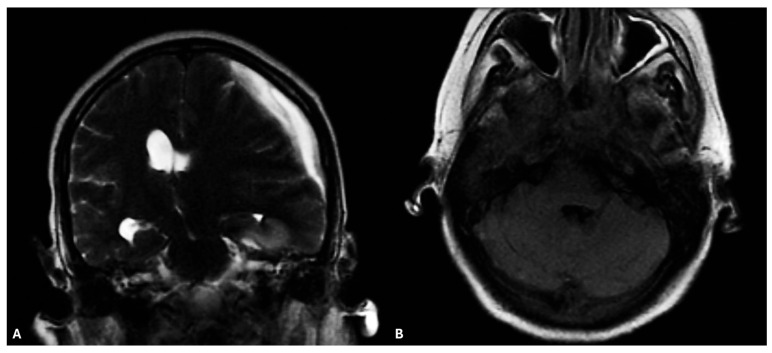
(A) Brain RM revealed Left hemispherical supratentorial subdural fluid layer, without hypointense hemorrhagic components in SWI in context, with a maximum thickness of 17 mm (vs. 14 mm), presenting with peripheral contrast enhancement and diffusion restriction, similar to empyema. Increased mass signs with compression of the left ventricle, and an 11 mm (vs. 9 mm) displacement of the septum pellucidum to the right, with apparent left uncial progression. Right ventricular dilation (due to incarceration), with T2W/FLAIR hyperintensity at the level of the posterior horn, in a context of hydrocephalus due to obliteration of the foramina of Monro. (B) Evaluation of the facial structures demonstrated extensive mucosal edema involving the sphenoidal sinuses and ethmoidal labyrinths, suggestive of an underlying sinonasal infection with possible contiguous spread to the intracranial compartment. No signs of acute intracranial hemorrhage or hydrocephalus were observed. These findings raised concern for cerebritis and incipient subdural empyema. Technique: 1.5 T scanner, FSPGR, FSE, SWAN, 3D-FLAIR, and DWI sequences in multiple planes before and after intravenous administration of paramagnetic contrast medium (ProHance 0.2 mL/kg).
